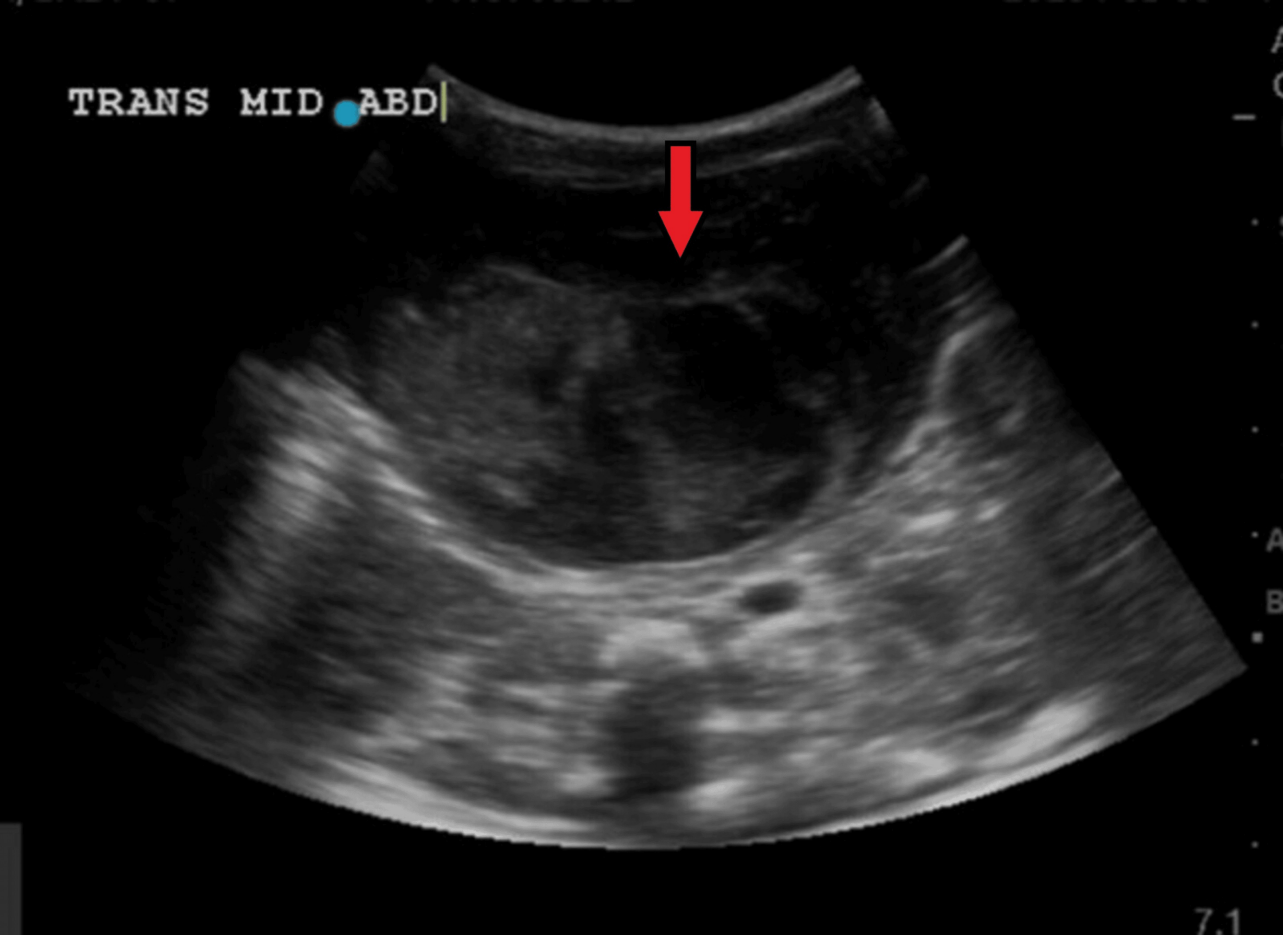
Abdominal ultrasound showed a large, complex cystic lesion occupying the mid-abdomen, more toward the right side.
